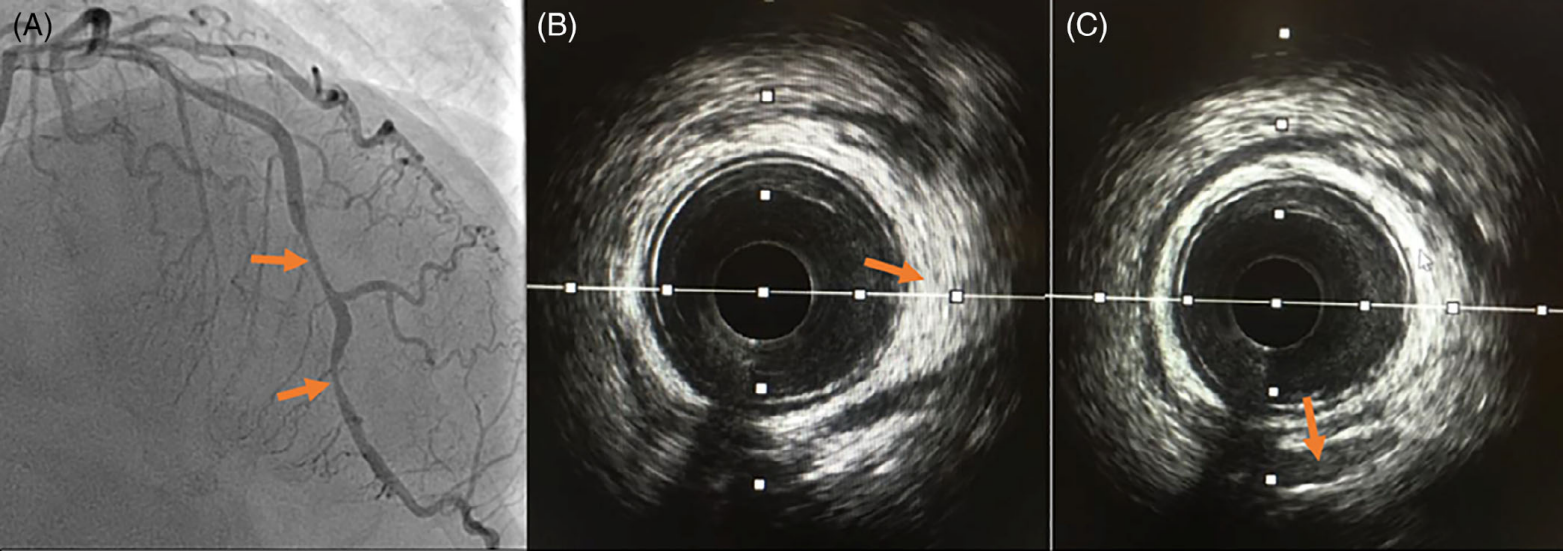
(A) Angiography showing narrowing of left anterior descending artery (arrowed). (B, C) Intravascular ultrasound of the same coronary artery showing intramural hematoma (echogenic signal) and dissection plane (echolucent signal) respectively, as depicted by arrows
